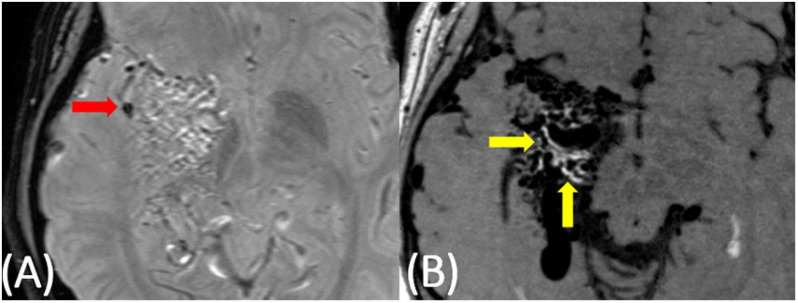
20-year-old female with a right temporoparietal AVM with a small area of sub-clinical evidence of prior hemorrhage on susceptibility-weighted imaging (A, red arrow). On post-contrast VW-MRI (B), there were multiple areas of hyperintensity at the vessel wall (yellow arrows).
